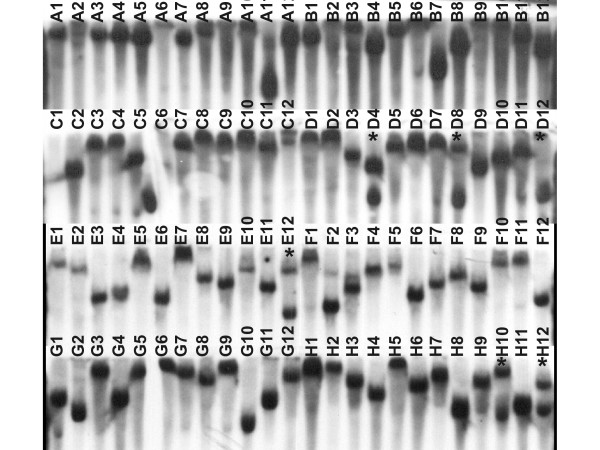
Southern blot analysis shows transposon isertion. X-ray film image after exposure to DNA of *Xanthomonas citri *subsp. *citri *strain 306 isolated mutant clones, previously cleaved with *Eco *RI and hybridized with the sequence of the transposon Tn5 labeled with the AlkPhos Direct RPN 3680 kit (Amersham Biosciences). Mutants with a double insert are marked with an asterisk.
